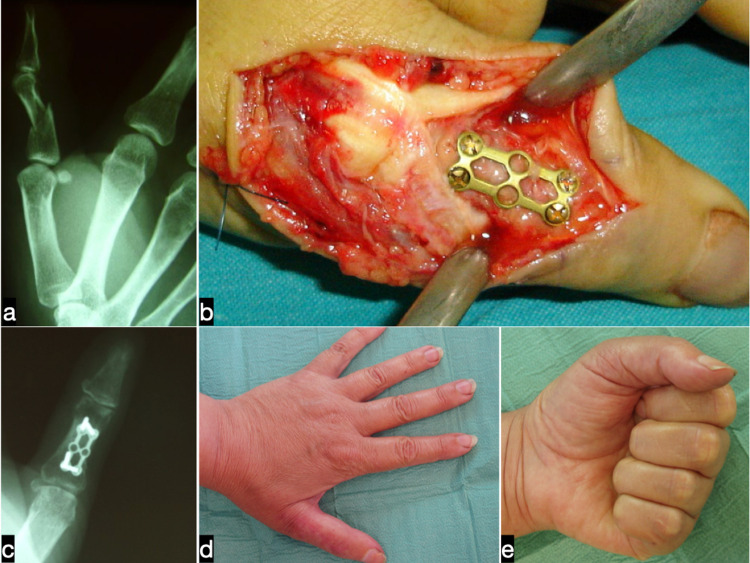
Preoperative (a) anteroposterior radiograph of a closed extraarticular fracture of the proximal phalanx of the dominant thumb in a 49-year old female patient. Intraoperative view of the fracture fixation with the use of a titanium H-plate (b). Radiographic (c) and clinical (d,e) outcome, six years postoperatively
